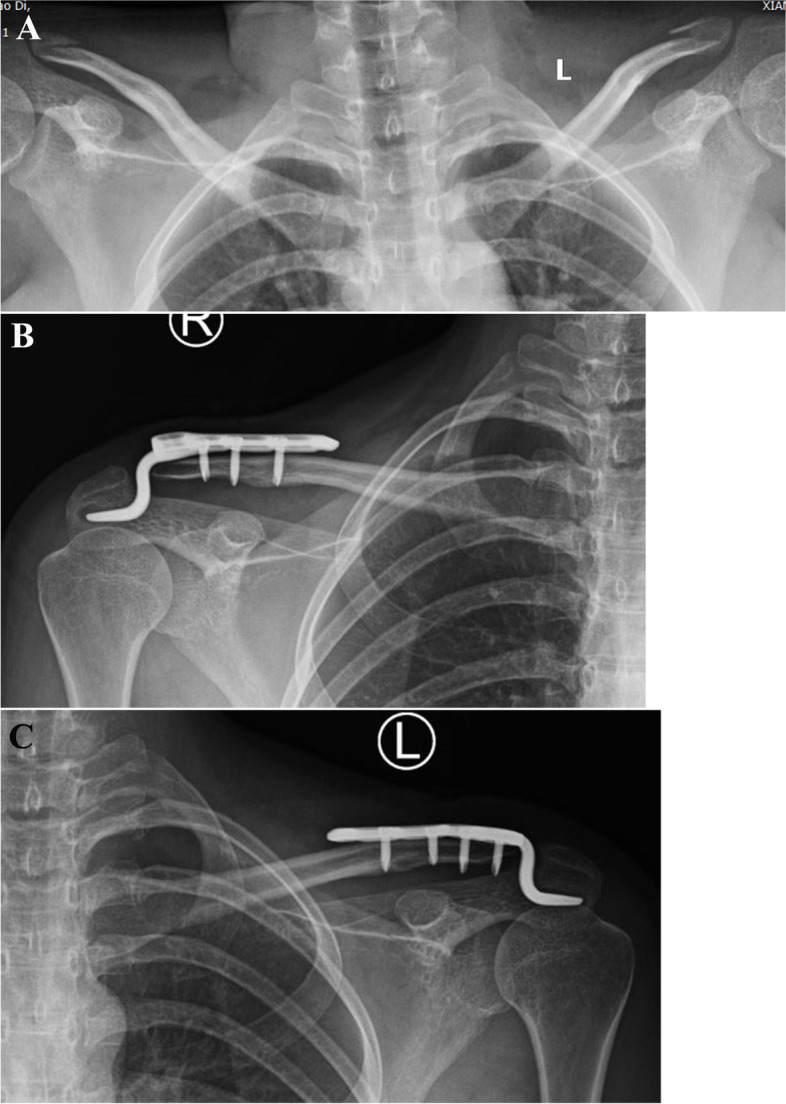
Radiographs of a 28-year-old woman who sustained bilateral clavicle fractures caused by a car accident; she also had a right radial shaft fracture, left ulnar olecranon fracture, and lumbar fracture. A Preoperative radiograph showed bilateral displaced distal clavicle fractures. The fractures on both sides were fixed with a hook plate. B, C Radiographs taken at the 1-year follow-up showed that the bilateral fractures had achieved bone union without implant migration
